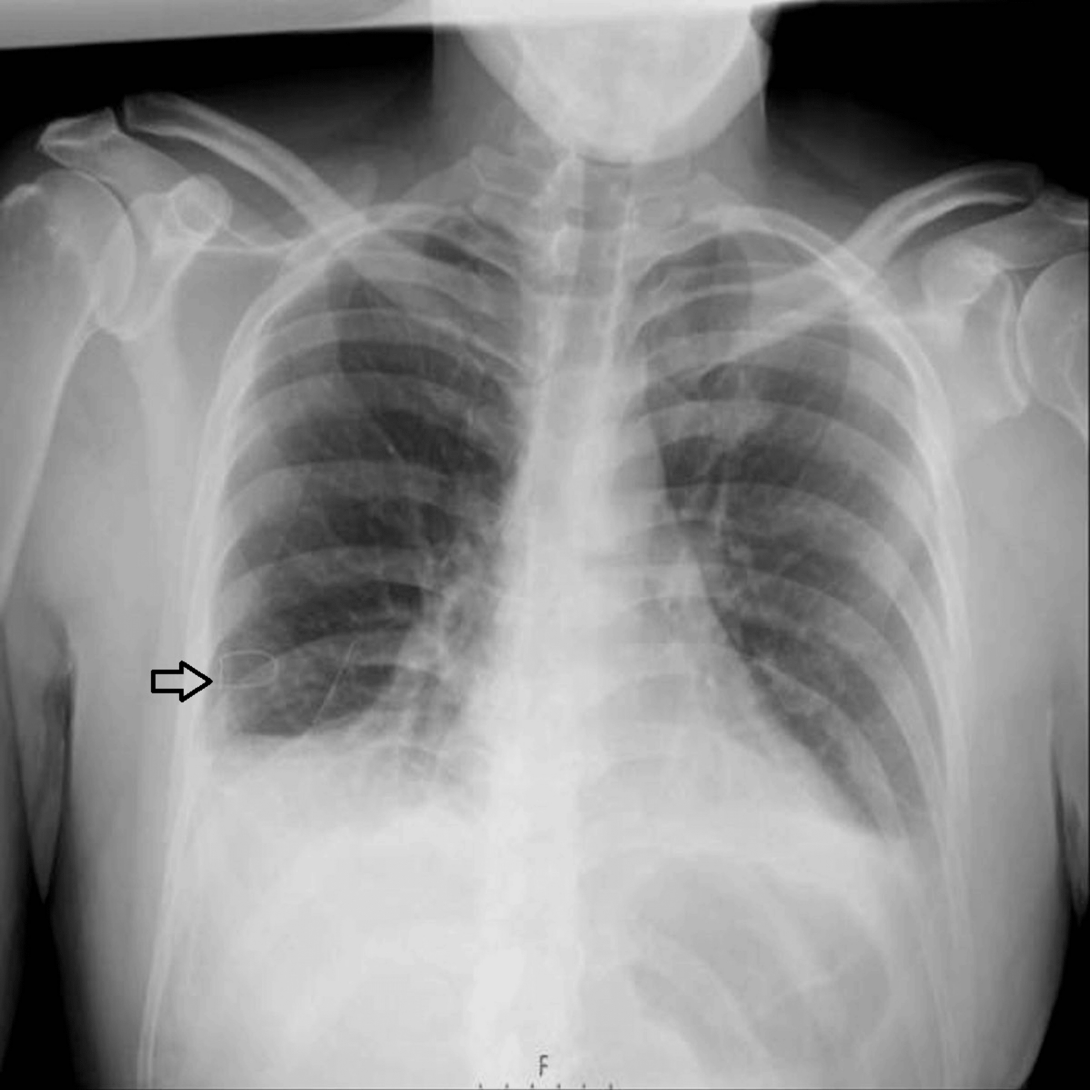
Chest X-ray after the insertion of the right-sided chest drain.
